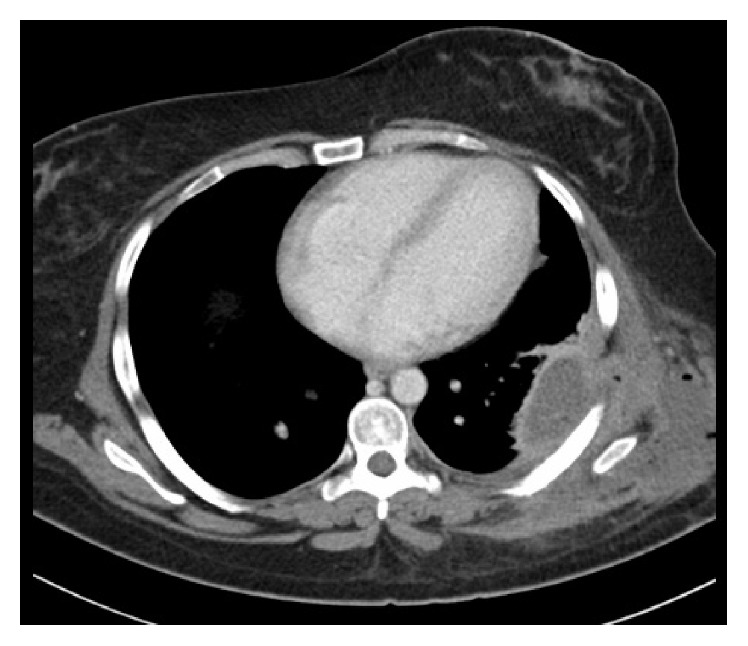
Axial CT scan of the chest demonstrating the intrapleural collection with pockets of air consistent with empyema.
